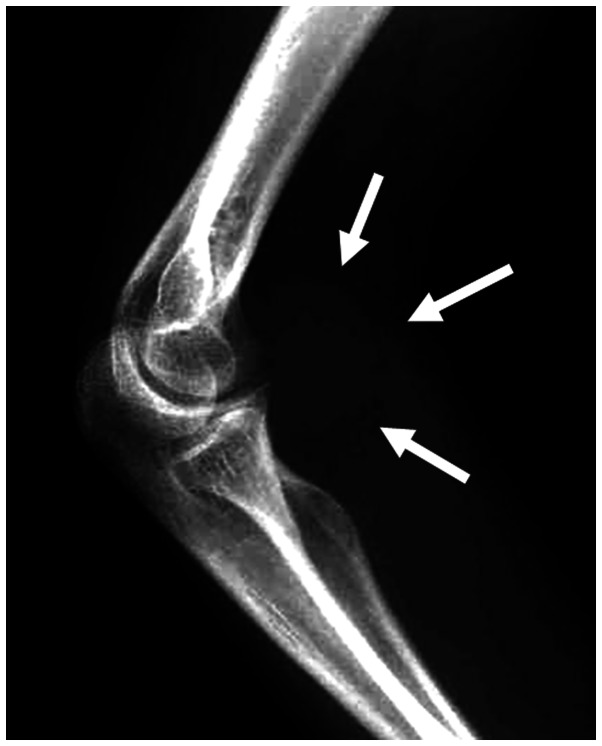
Radiograph showing a soft tissue mass (arrow) on the right elbow, with no apparent bone erosion.
